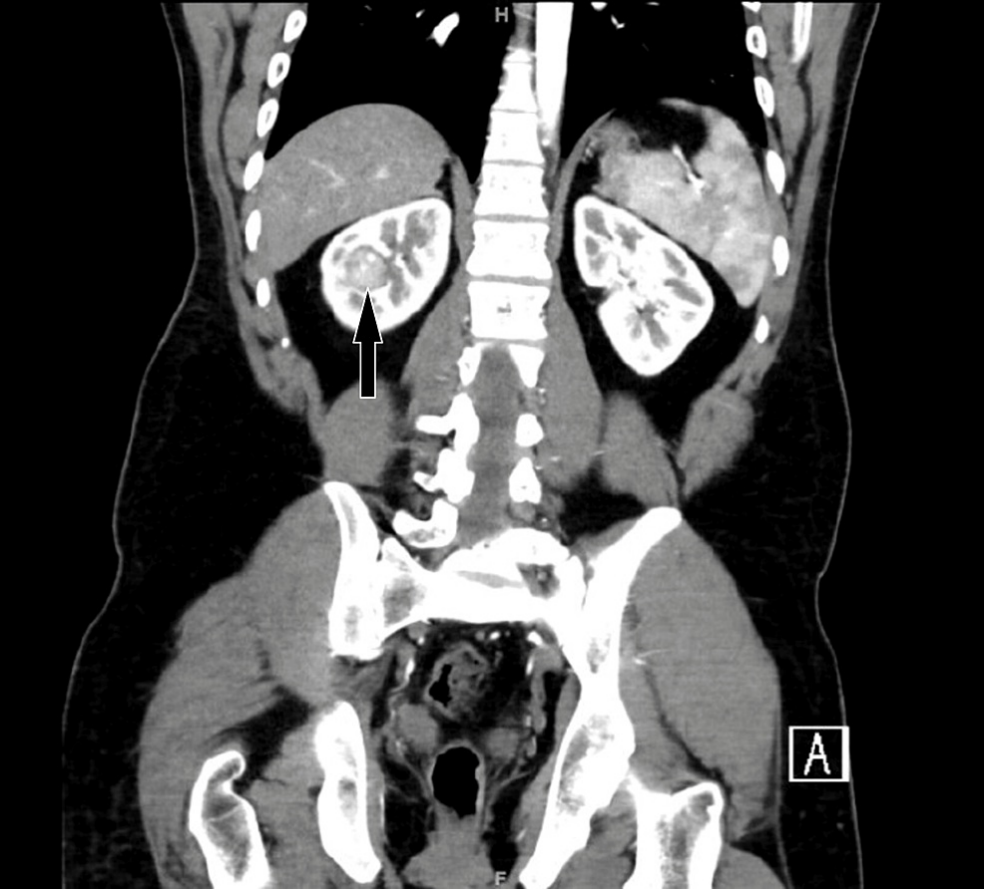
Contrast CT scan of the abdomen and pelvis, coronal slice There is a right renal mid to upper pole enhancing renal mass measuring 1.8 x 2 x 2.2 cm.
